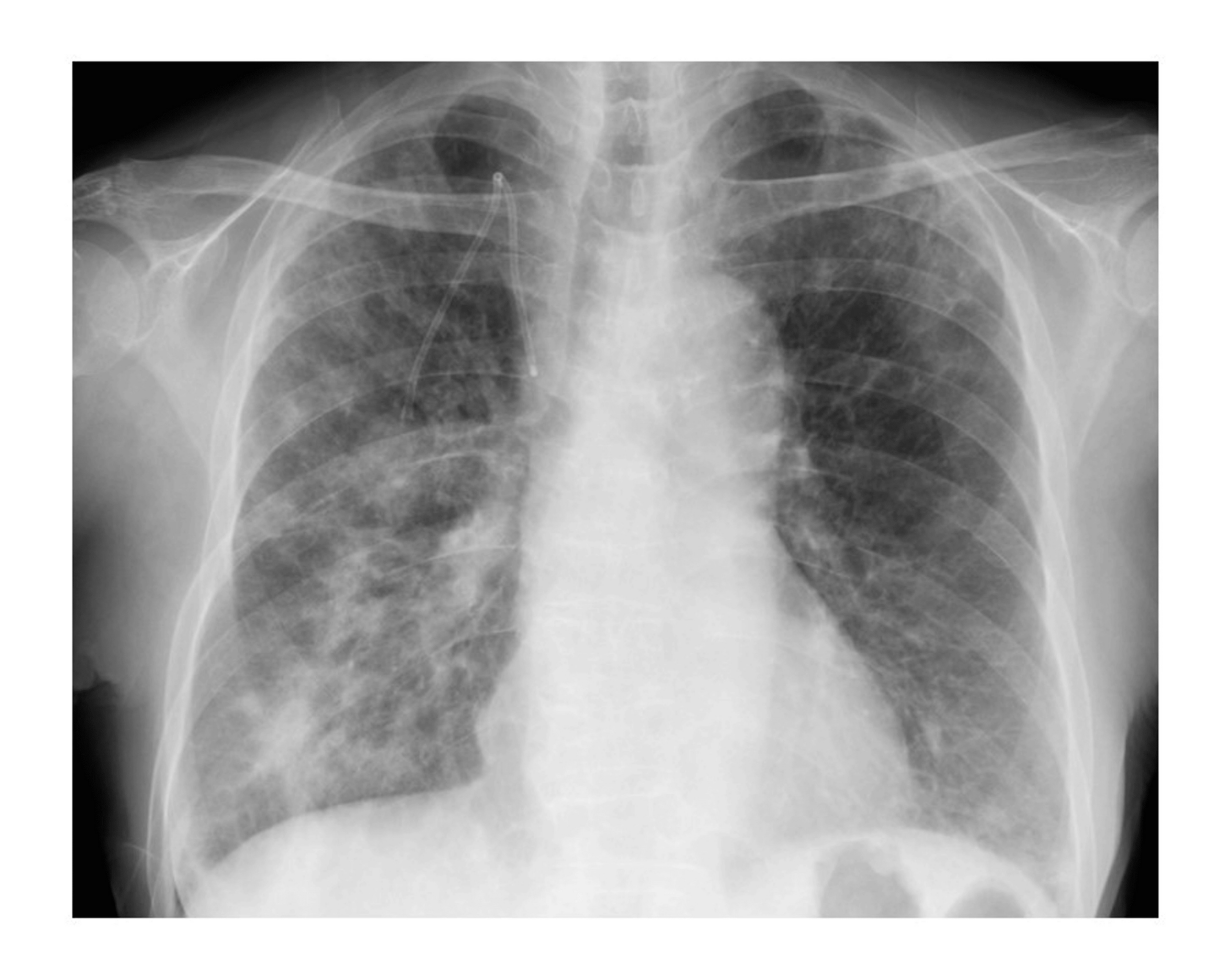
Chest X-ray after five cycles of pembrolizumab
